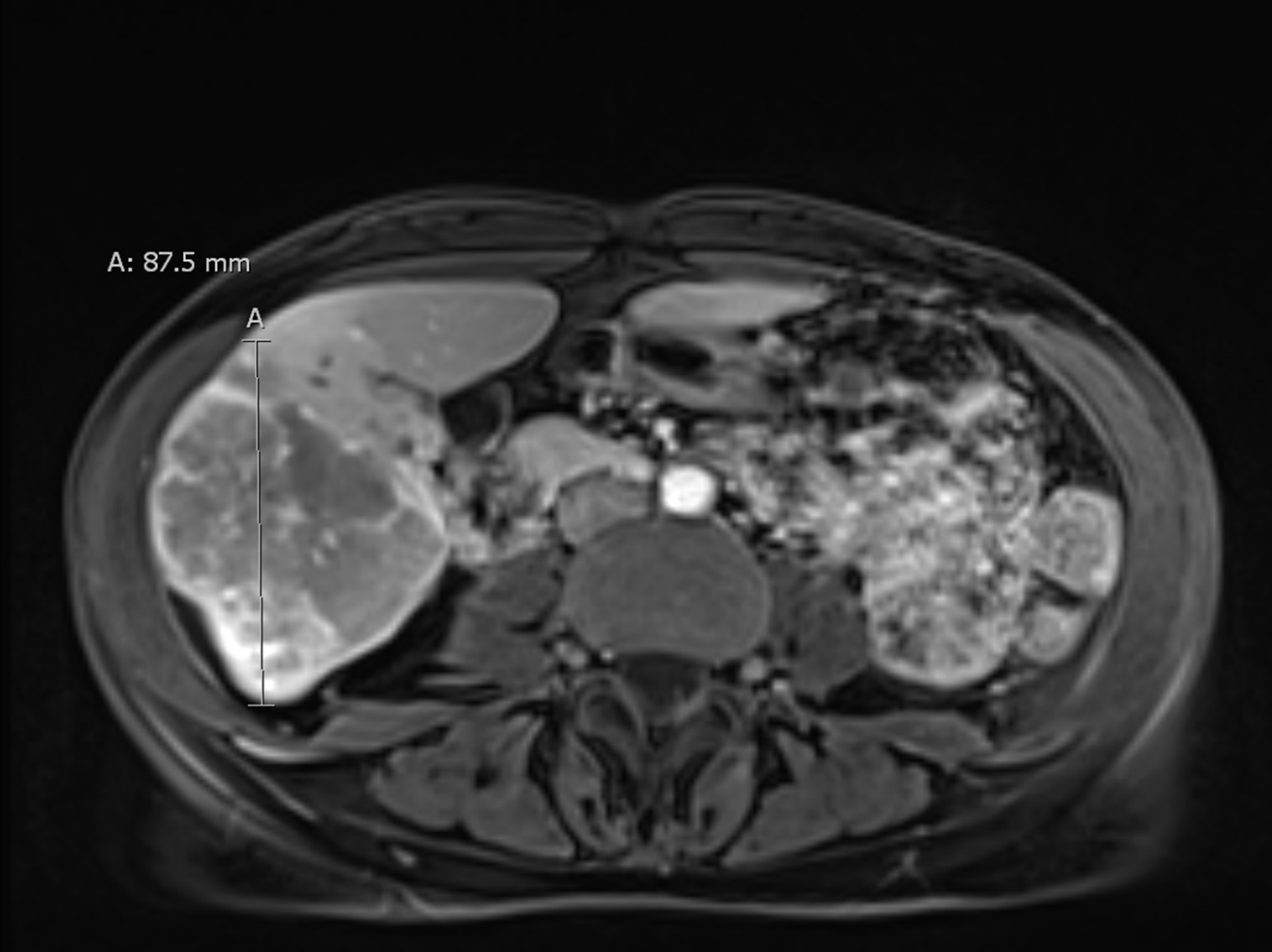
Representative axial slice from pre-treatment MRI
